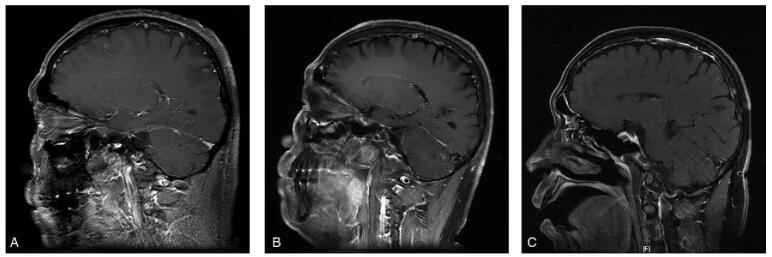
Head MRI imaging results for the case 2 during treatment with dacomitinib. (A) Brain MRI revealed 2 abnormal nodular enhancements in the left frontal lobe at diagnose (April 20, 2020). (B) Three months after treatment, brain MRI showed disappearance of the intracranial metastases, which was judged as a CR. (C) MRI indicated 2 nodules of abnormal enhancement in the right frontal lobe at the time of disease progression (January 7, 2021). CR = complete response, MRI = magnetic resonance imaging.
